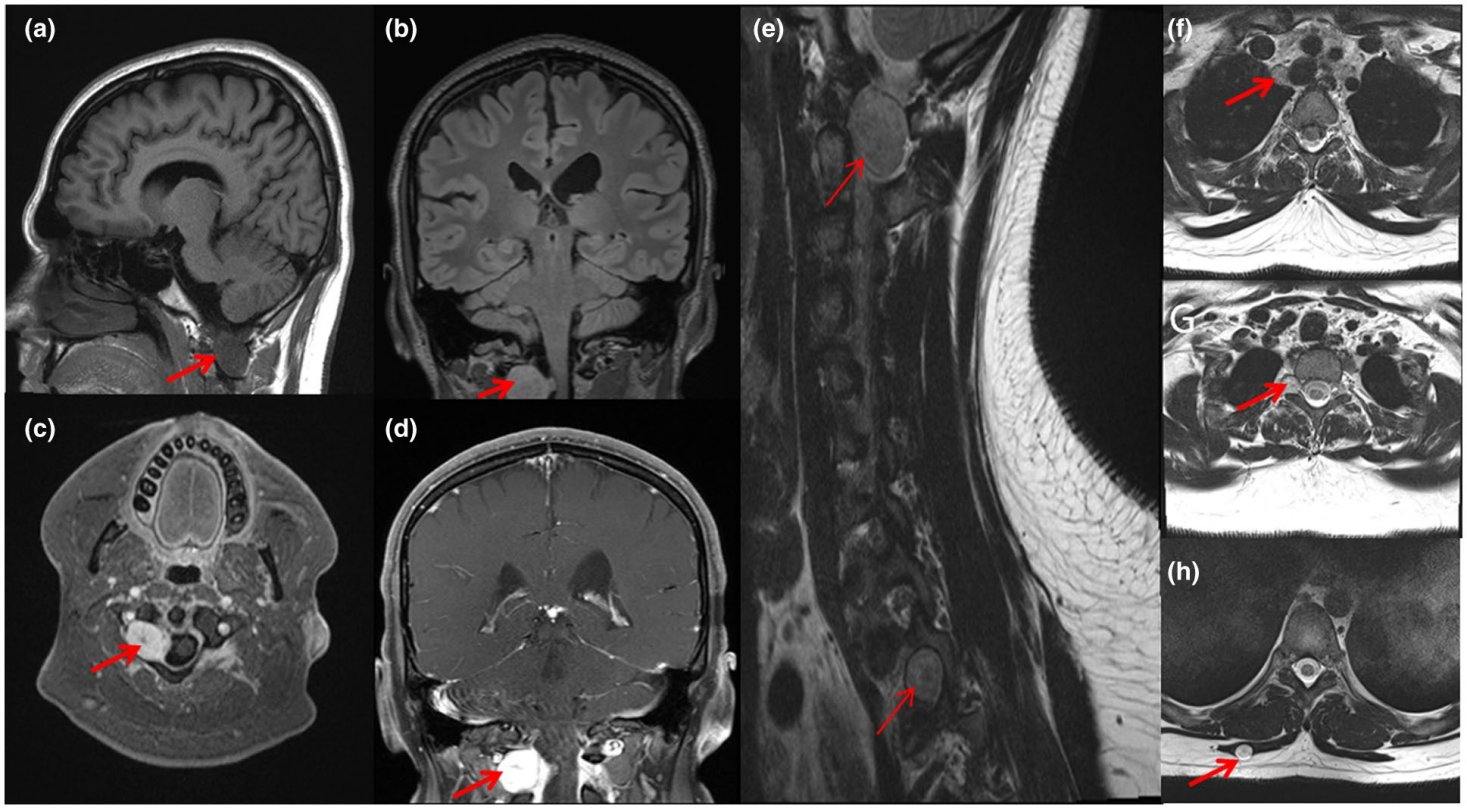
MRI Brain images (a–d) showing T1 hypointense (a), FLAIR isointense (b), and homogenously enhancing lesion (c & d) (arrows) in right C1–C2 region. MRI T2 sagittal section of cervicodorsal spine (e) showing two lesions, one at C1–C2 and the second at T1–T2 (arrows). Also note lesions in T2 axial images visualizing a lesion (arrows) in right superior mediastinum (f), right T1–T2 neural foramen (g), and right paraspinous muscle (h)
